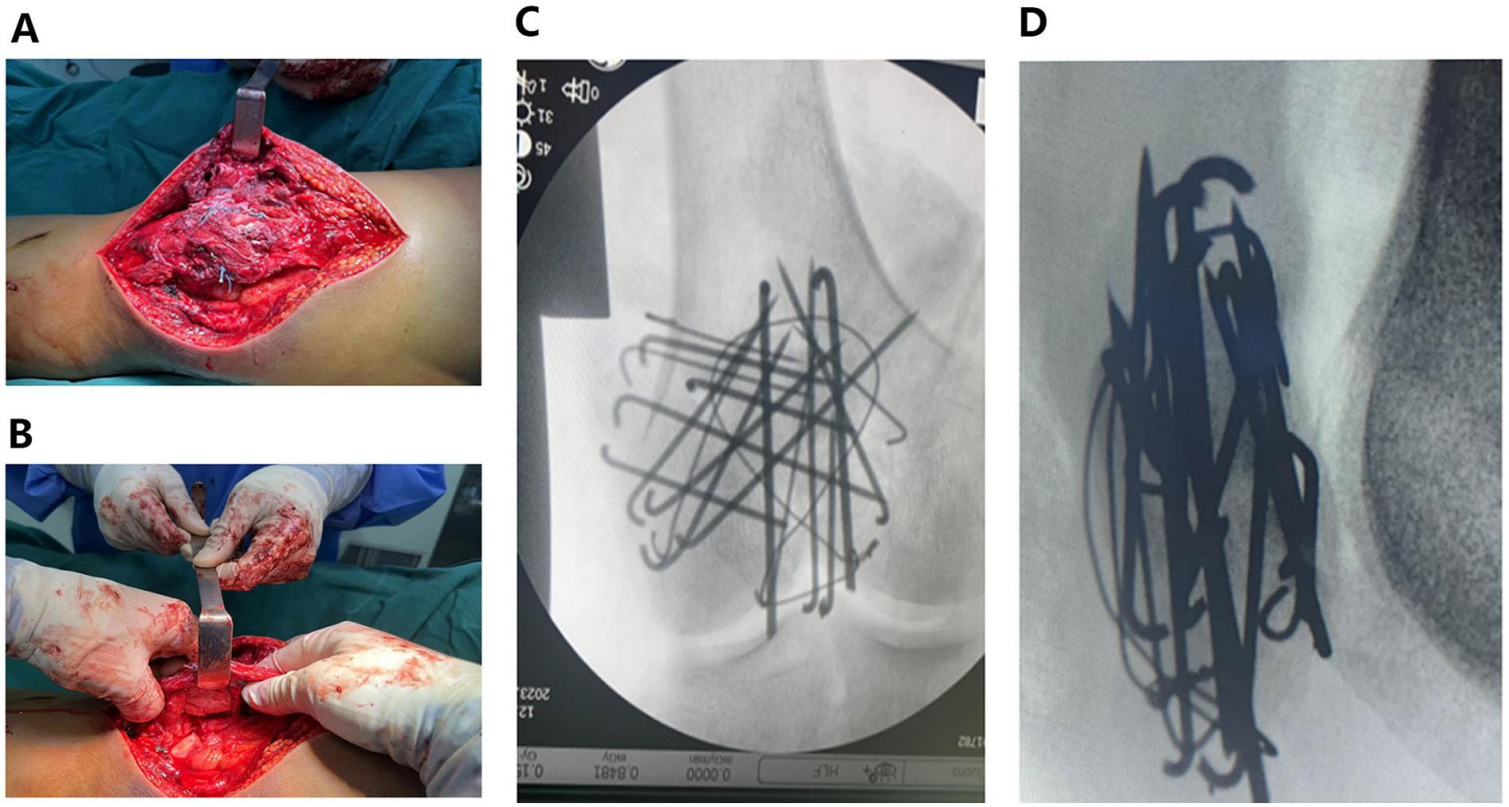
(A) The periosteum on the surface remains intact. (B) The articular surface is anatomically reduced. (C,D) Anteroposterior and lateral x-ray fluoroscopy images indicating that internal fixation is appropriate and that the degree of reduction is satisfactory.
